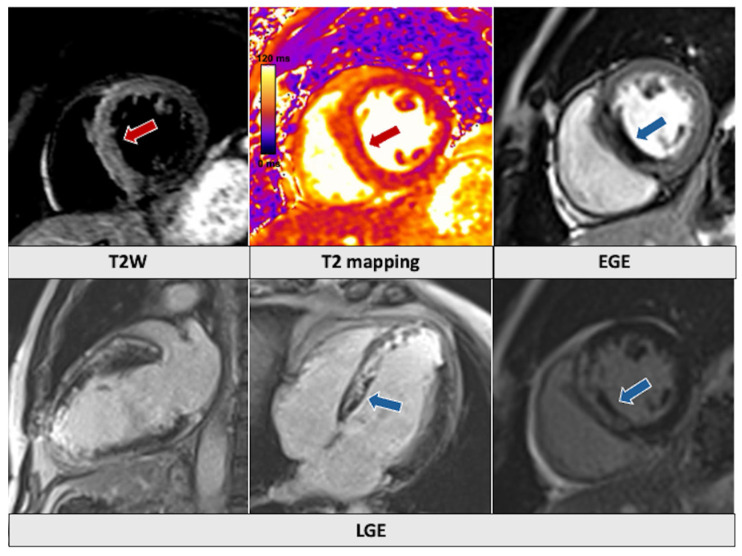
Patient admitted with anterior ST-elevation myocardial infarction. Cardiac magnetic resonance images showed evidence of increased signal intensity in T2-weighted images and increased T2-mapping values in the interventricular septum and anterior wall (red arrows). There is also evidence of microvascular obstruction in both early and late gadolinium enhancement images (blue arrows,) along with transmural LGE in the septum and apical segments. LGE: late gadolinium enhancement.
